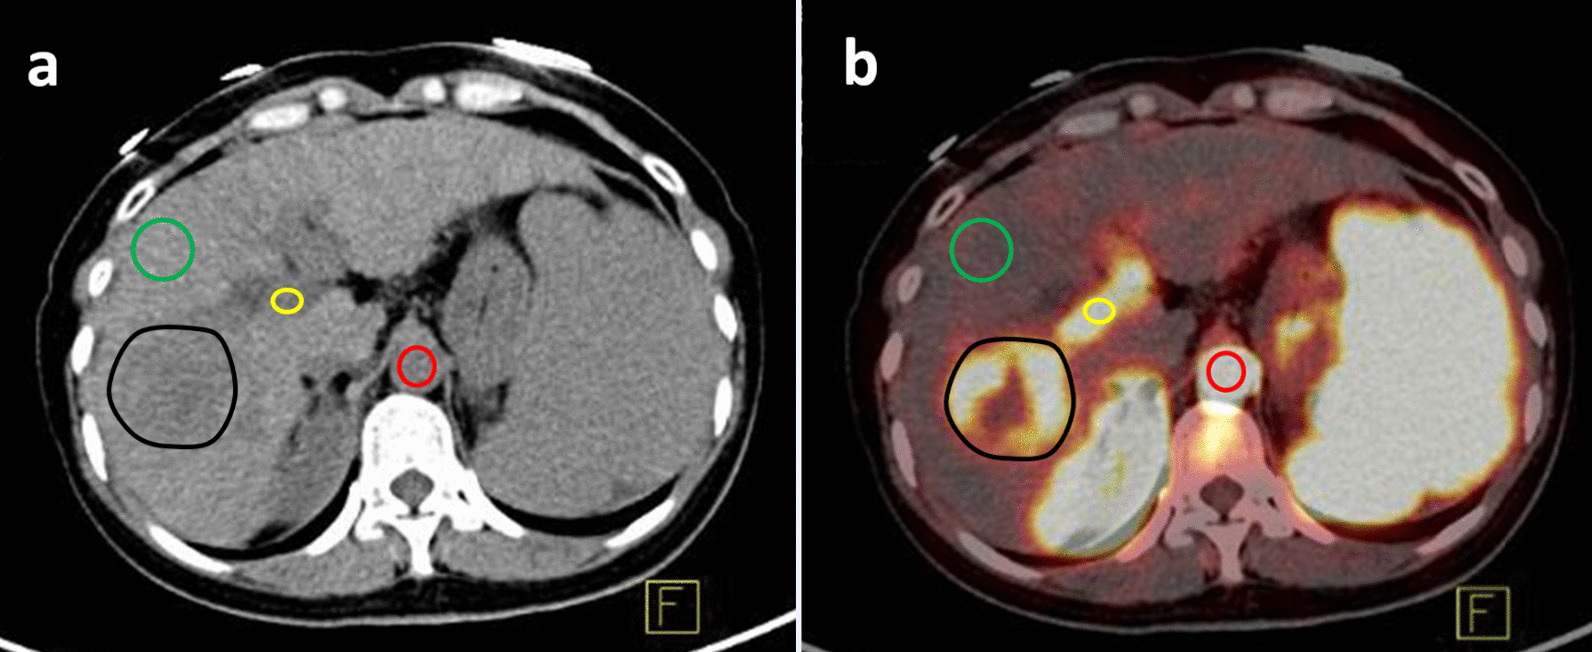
Region of interest drawn in dynamic PET/CT. a CT image, b PET/CT fusion image. The HCC is shown in the black circle, background liver tissue is shown in the green circle, the portal vein is shown in the red circle, and the aorta is shown in the yellow circle. Blood ^18^F-FDG enters the aorta, portal vein, spleen and HCC
